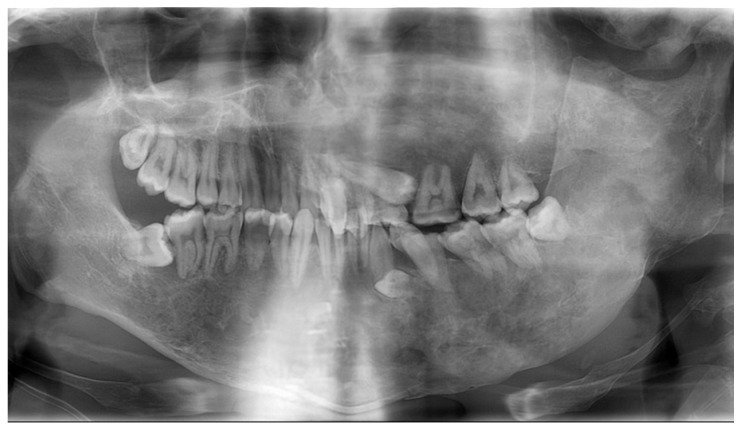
Panoramic radiograph at the age of 29 years and 10 months.
